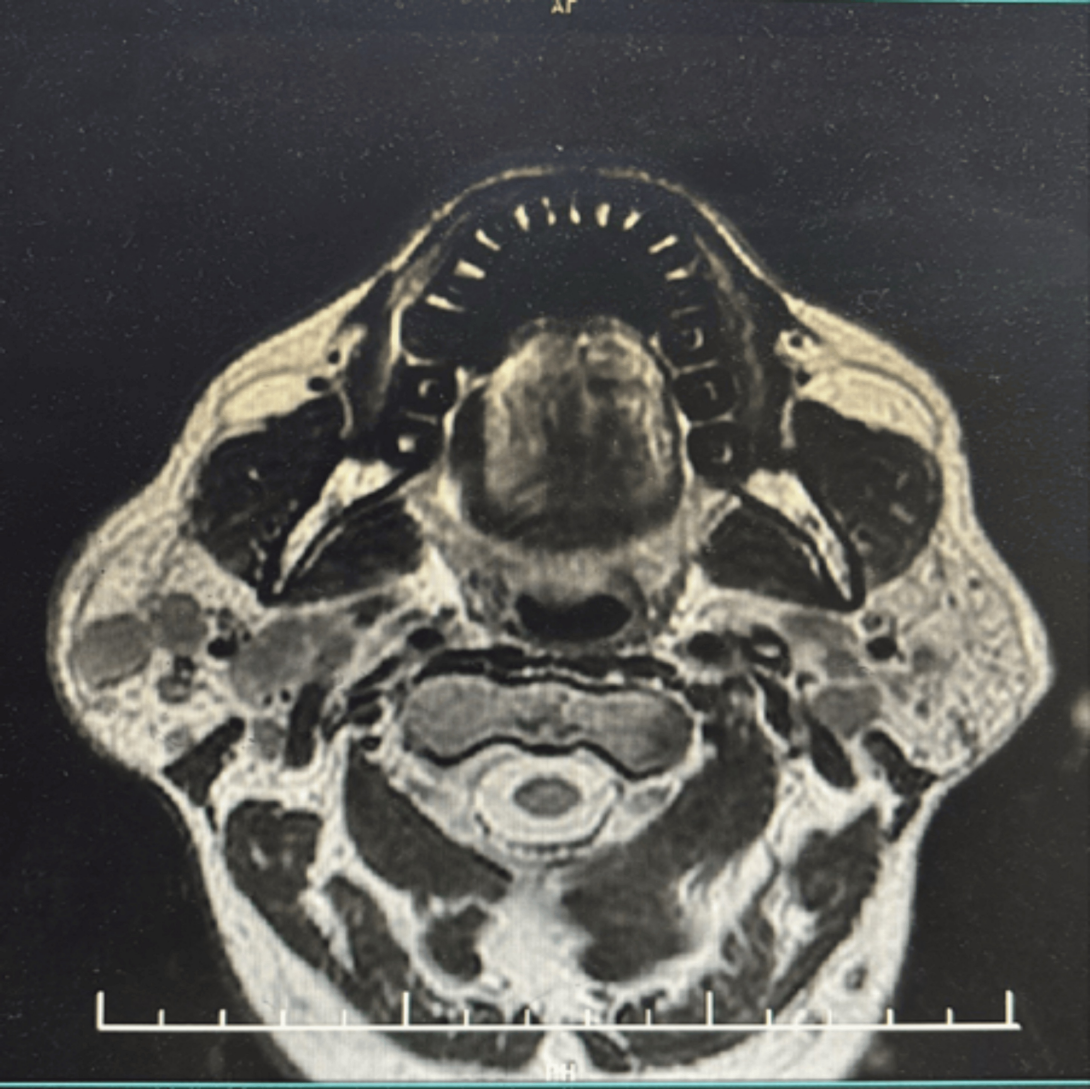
Axial T2WI of the patient The axial T2-weighted image (T2WI) shows multiple T2 hypo- to isointense lesions in the right parotid gland.
